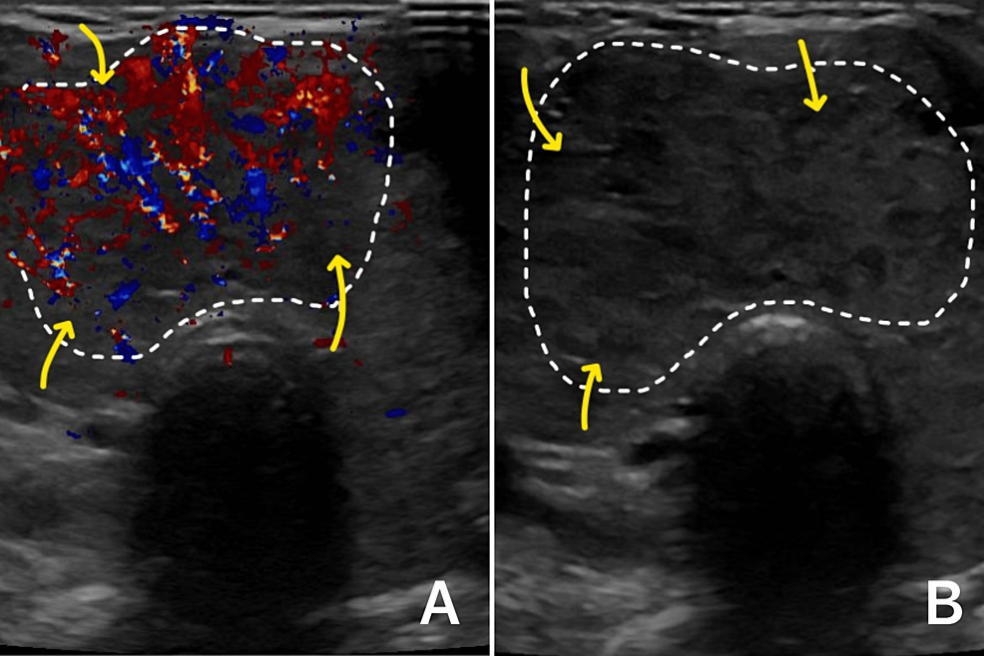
Color Doppler ultrasound of the neck (A) and transverse gray‑scale ultrasound (B) (A) The image demonstrates both central and peripheral hypervascularity of the thyroid gland (yellow arrows). (B) The image shows a diffusely enlarged, heterogeneous, and hypoechoic thyroid mass (yellow arrows), making the diagnosis of Graves' disease more likely
